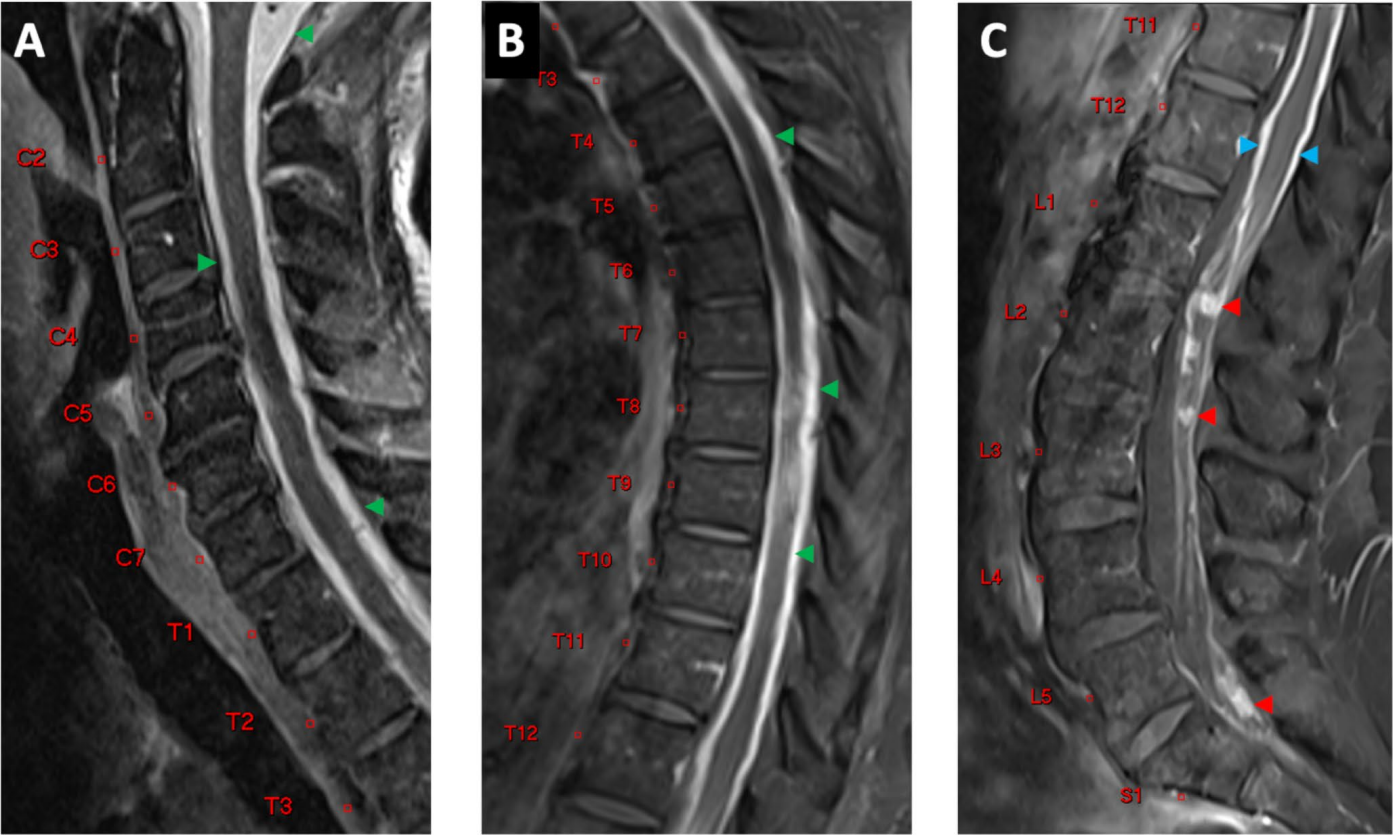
MRI Spine. Sagittal T1-weighted postcontrast MRI of the Cervical (A), Thoracic (B), and Lumbar (C) spine. Sagittal T1-weighted postcontrast imaging of the cervical and thoracic spine demonstrates diffusely hyperintense subarachnoid spaces within the foramen magnum and intraspinal compartments (green arrowheads in A and B), which reflects a combination of extensive leptomeningeal tumor deposits and associated leakage of gadolinium contrast agent into the subarachnoid space. Sagittal t1-weighted postcontrast imaging of the lumbar spine demonstrates both smooth (blue arrowheads in C) and nodular (red arrowheads in C) leptomeningeal enhancement of the conus surface and cauda equina, respectively
